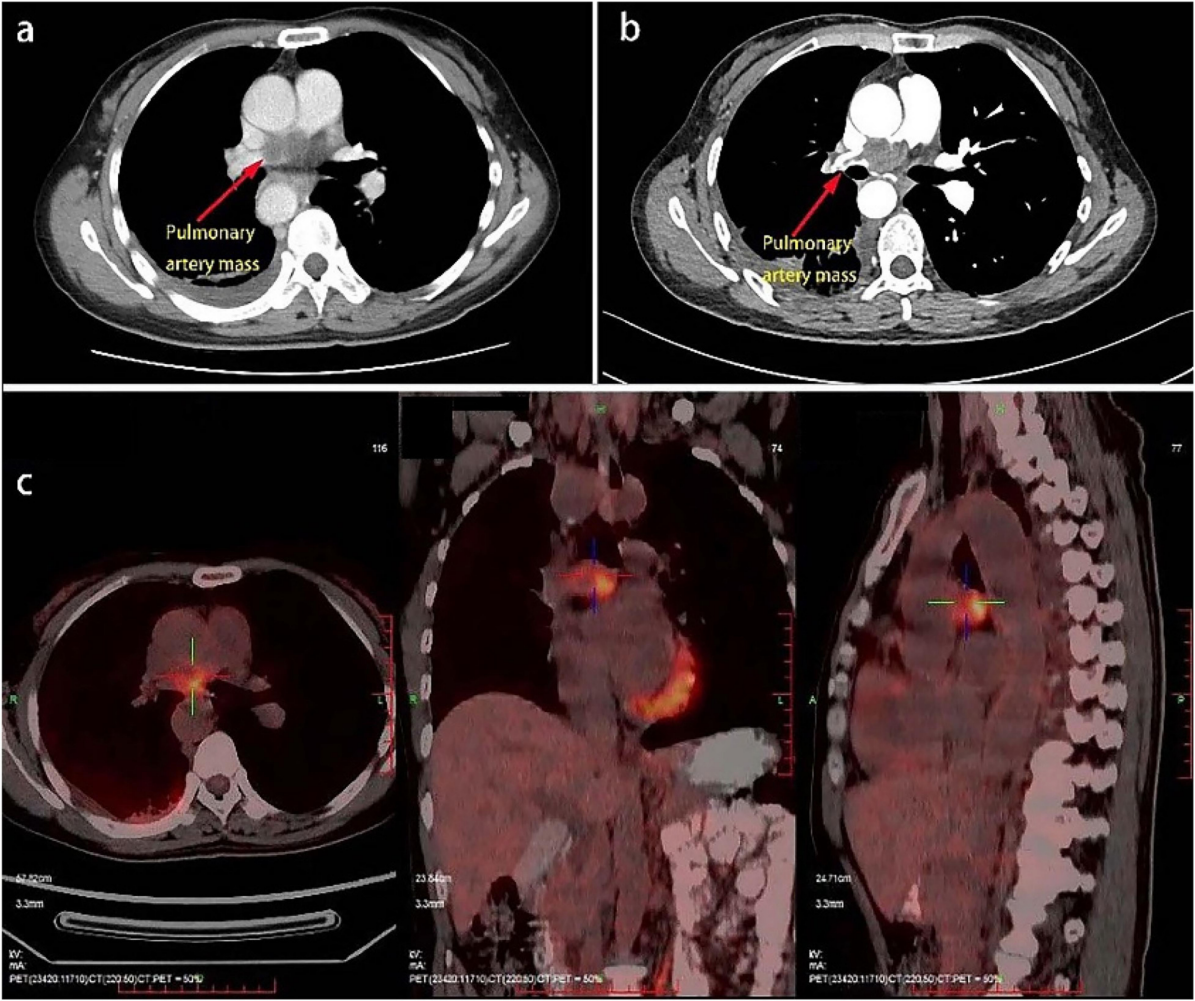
(a) chest CT shows an irregular filling defect in the right pulmonary artery trunk; (b) CTA of the thoracoabdominal aorta reveals a filling defect in the right pulmonary artery trunk, with involvement of the right upper lobe artery and right interlobar artery, distal narrowing, and sparse branching; (c) PET-CT demonstrates a patchy low-density shadow in the right pulmonary artery, measuring approximately 2.3*1.8*1.4 cm, with increased FDG uptake (SUVmax ∼10.0). No significant enlargement is observed in the mediastinal or bilateral hilar lymph nodes.
